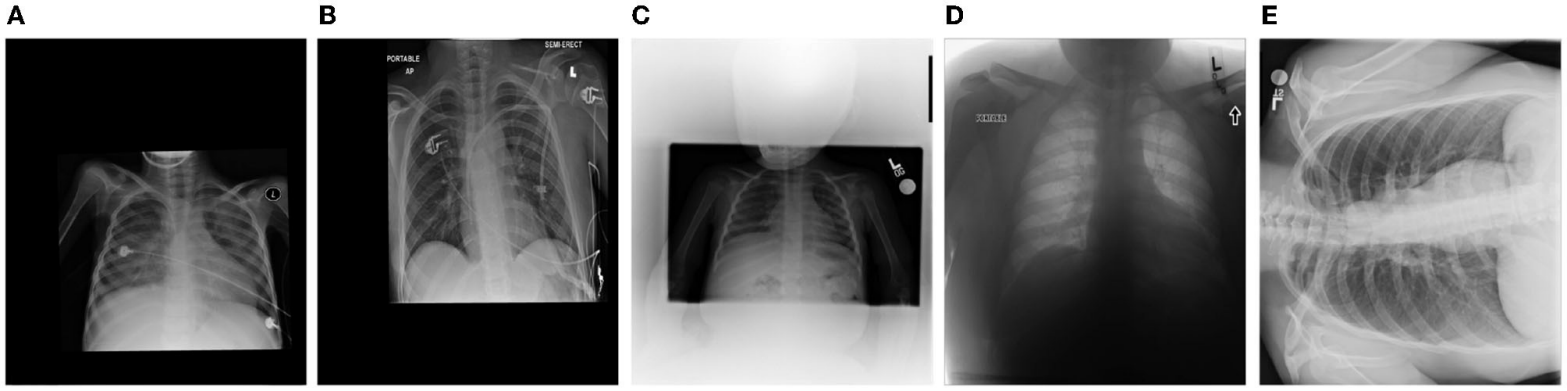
Example chest X-ray images (labeled A–E) with different types of significant distortions and visual anomalies from the Radiological Society of North America (RSNA) Pneumonia Challenge dataset.
